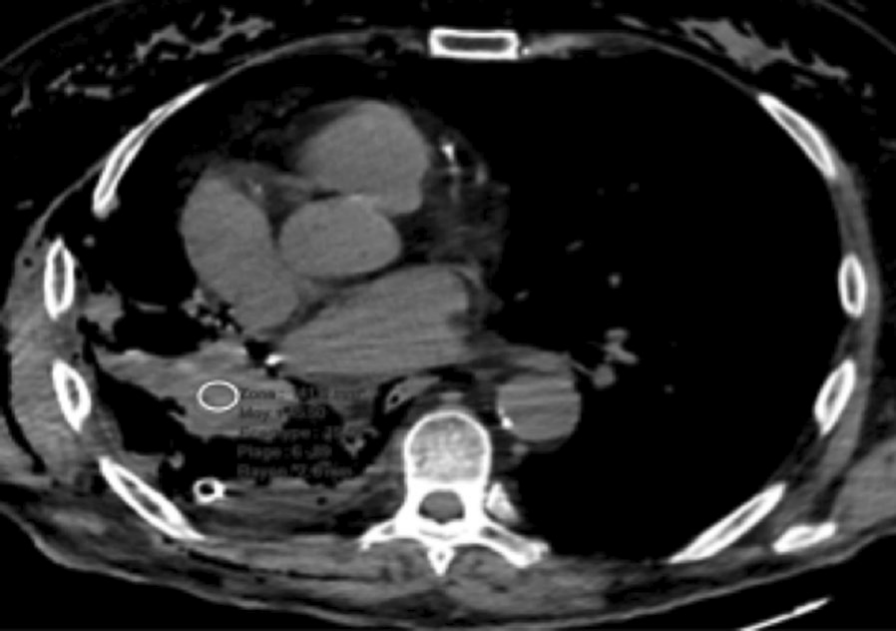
Haemothorax. Axial CT image (mediastinal window) showing haemothorax after right pneumonectomy. A region of interest placed in the effusion indicates a spontaneous density around 50 HUF.
